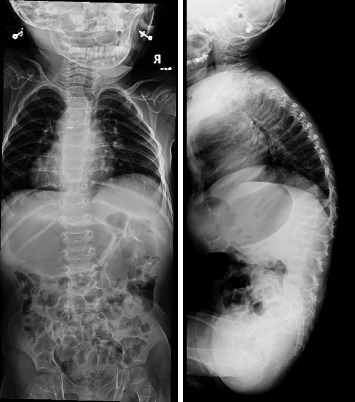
Radiographs at 1.75 years of age.
